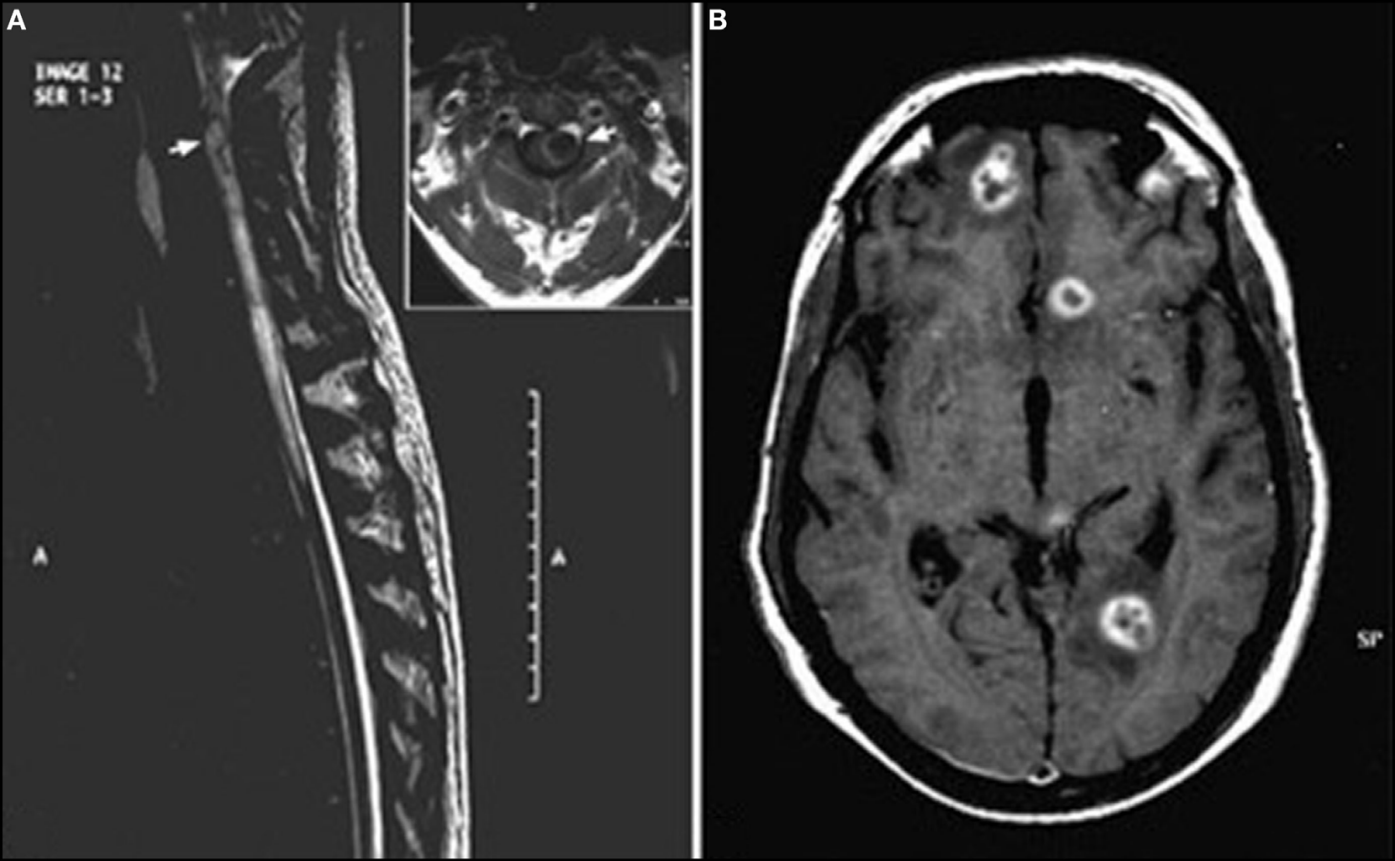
Encephalomyelitis (50) (A) T2-weighted sagittal image of spine showing a hyperintense lesion (arrow) with extensive perilesional edema in the upper cervical cord. Inset highlights large lesion (arrow) with peripheral hyperintense rim and perilesional edema causing expansion of cervical cord. (B) T1-weighted axial post-contrast image of brain shows multiple ring-enhancing lesions in bilateral frontoparietal deep white matter with nodular enhancement of the wall seen in left frontal lesion. This image has permissions for use.
